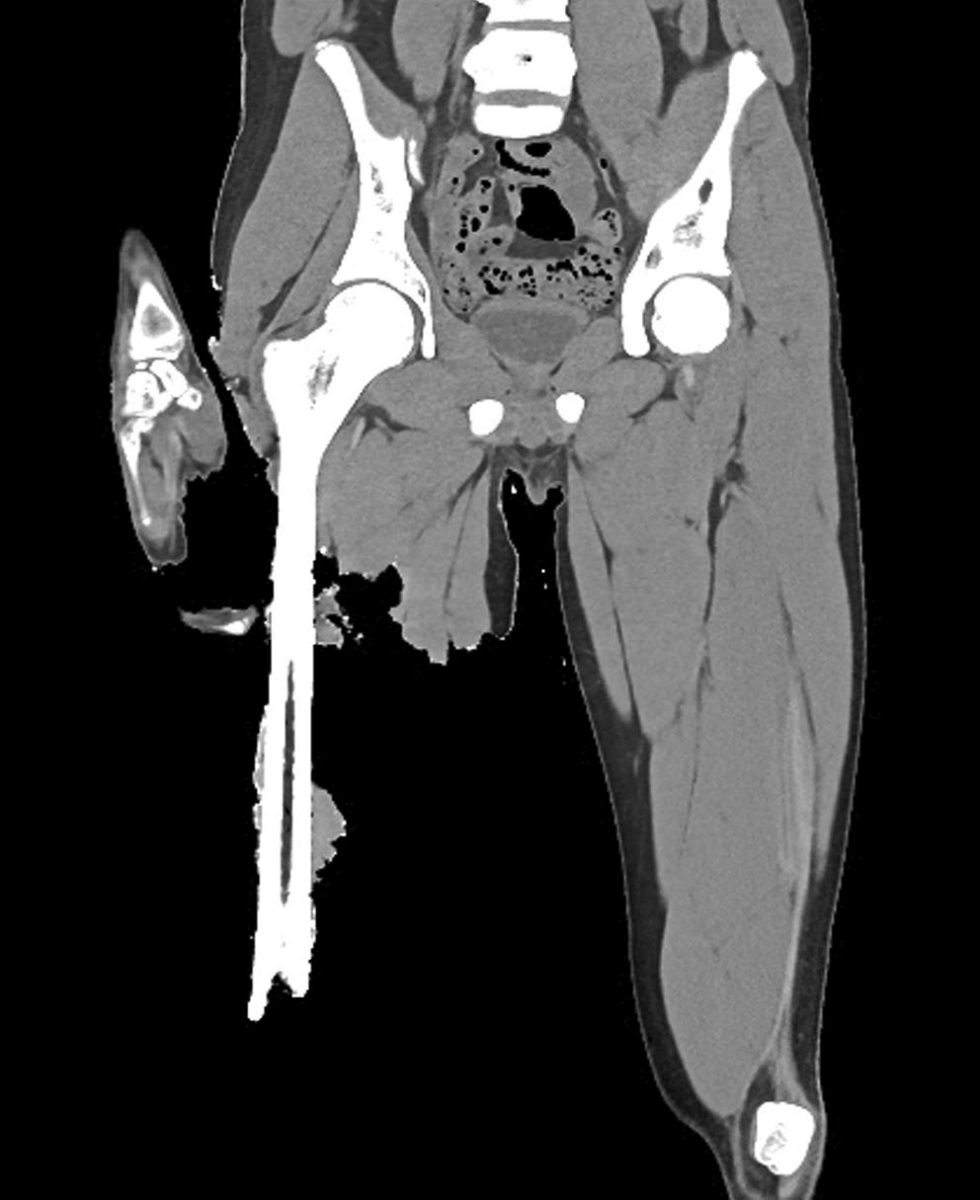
A post mortem CT scan showing loss of the distal femur and lower right leg along with extensive skin and soft tissue debridement
